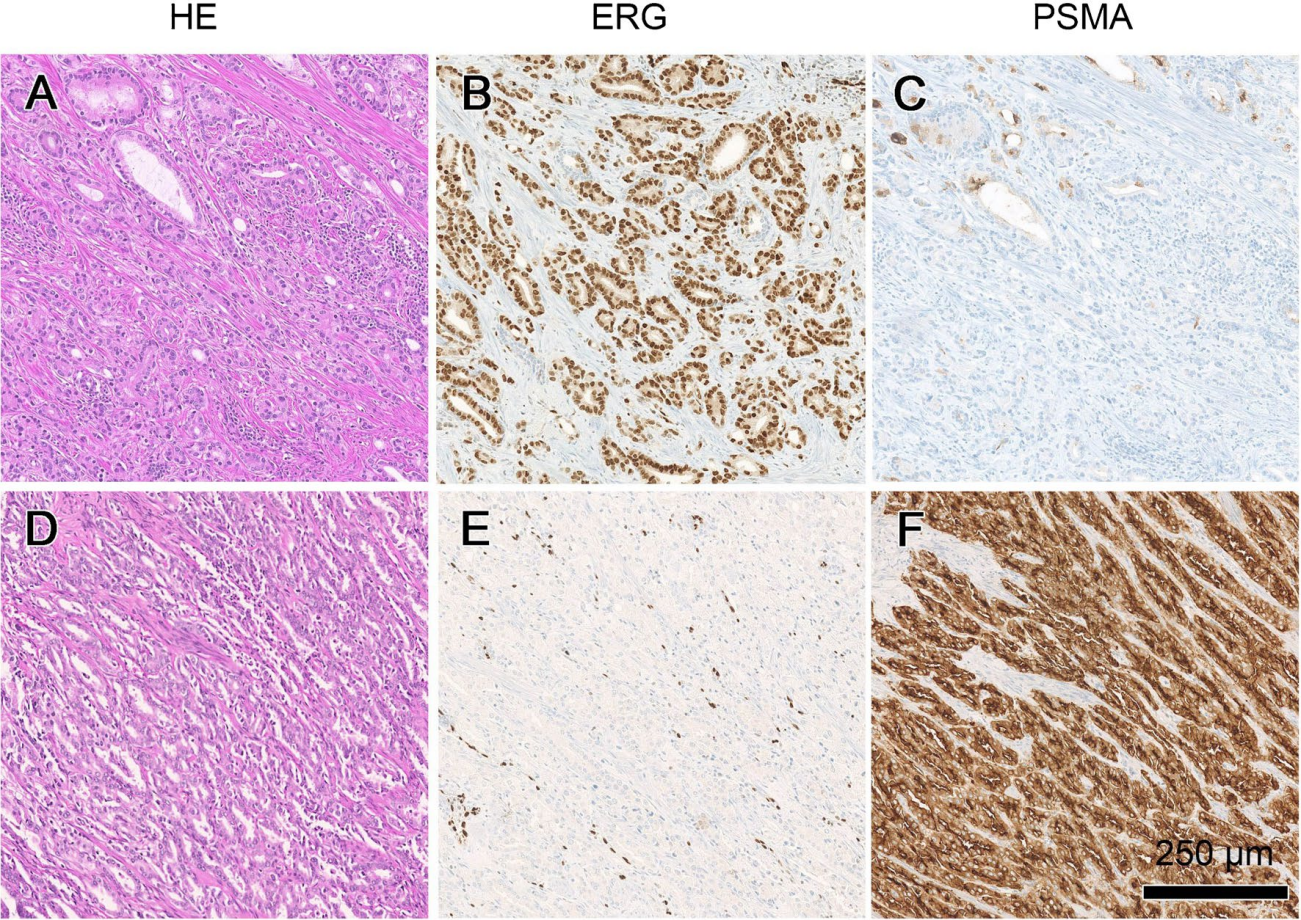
Overview of two prostate adenocarcinoma cases differentially expressing ERG and PSMA. The first case (A) shows nuclear ERG reactivity in the tumour cells (B), and vastly negative PSMA expression (C). The second case (D) depicts no ERG expression in the tumour (E) with internal endothelial positive control, and diffuse PSMA reactivity (F). Scale bar: 250 μm
